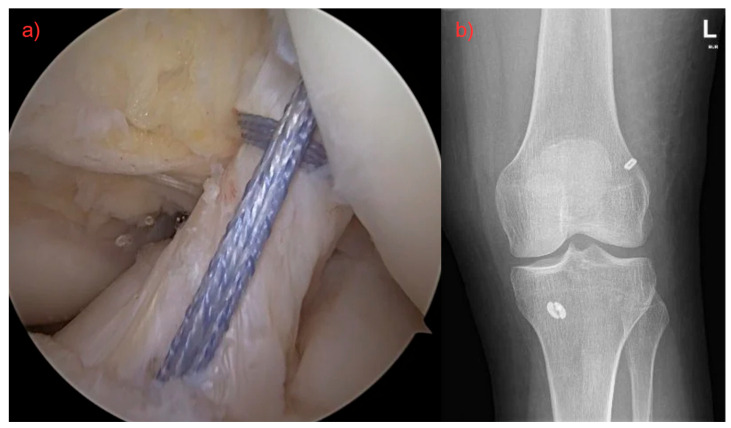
(a) Surgery of ruptured ligament by using FiberLink (Arthrex©), both fibers were pulled through the femoral canal, tightened, and fixed with an anchor. Final result with definitive ACL restoration. (b) Postoperative X-ray.
